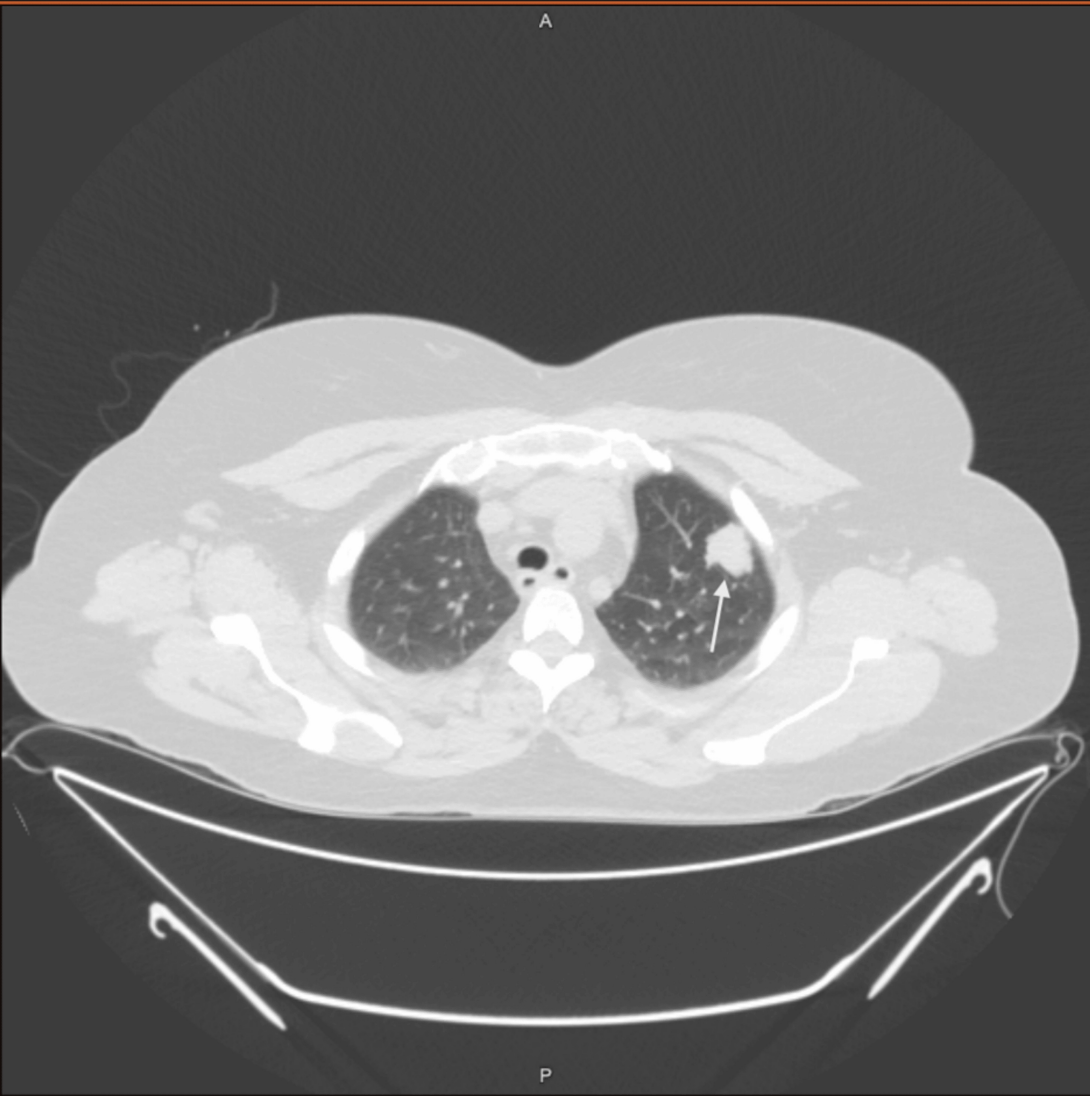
CT scan of the chest showing a 2×1.9 cm nodule in the upper lobe of the left lung (as highlighted by the arrow) CT: computed tomography
